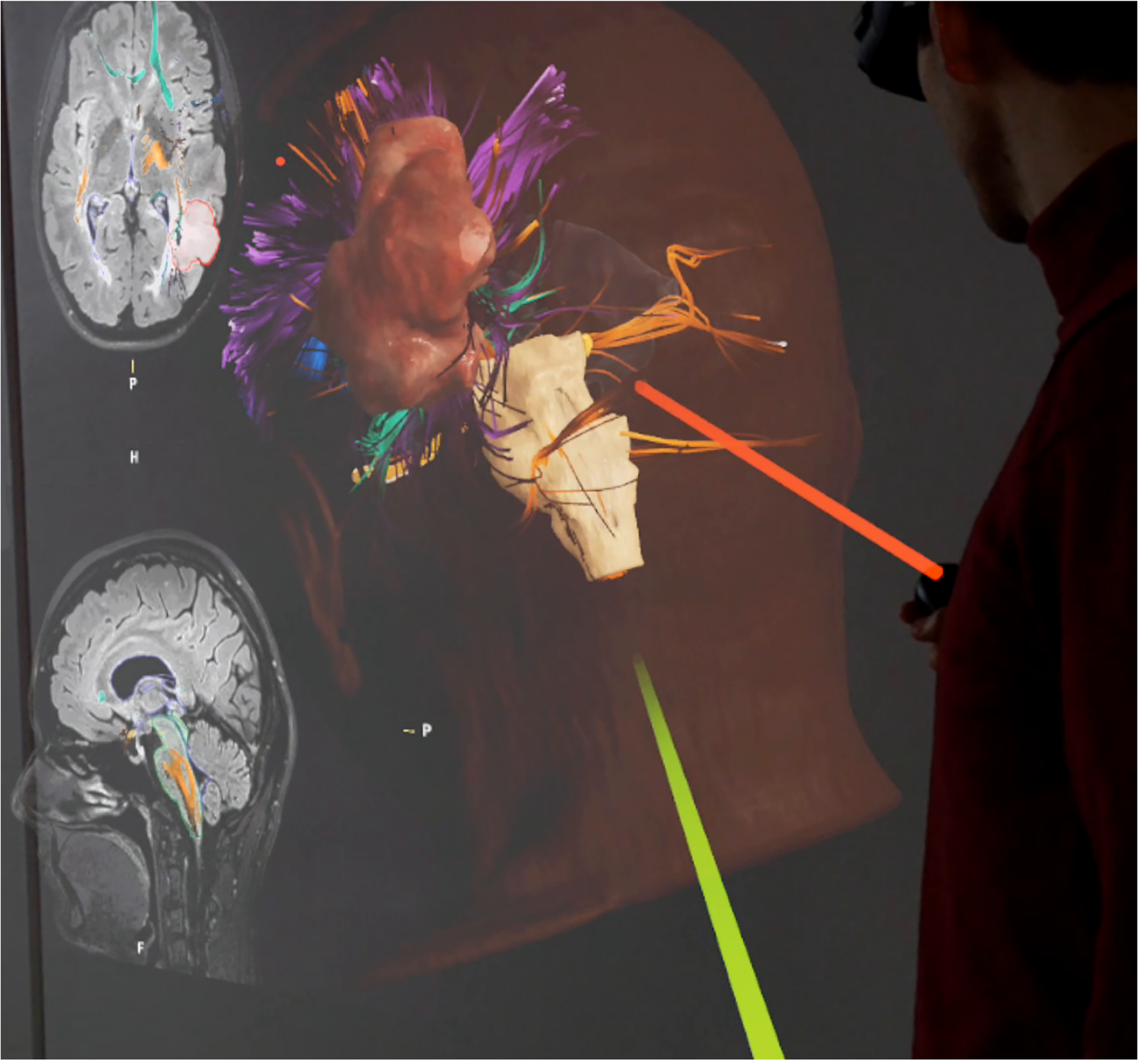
The figure shows a screenshot visualizing the participant view during the application of AR for fiber dissection. The 3-D reconstruction is shown from left occipital. The green and red laser pointers can be used to describe structures or answer questions between participants or moderator. Additionally, the patient’s 2-D MRI scan slices can be visualized in the background and can be scrolled
